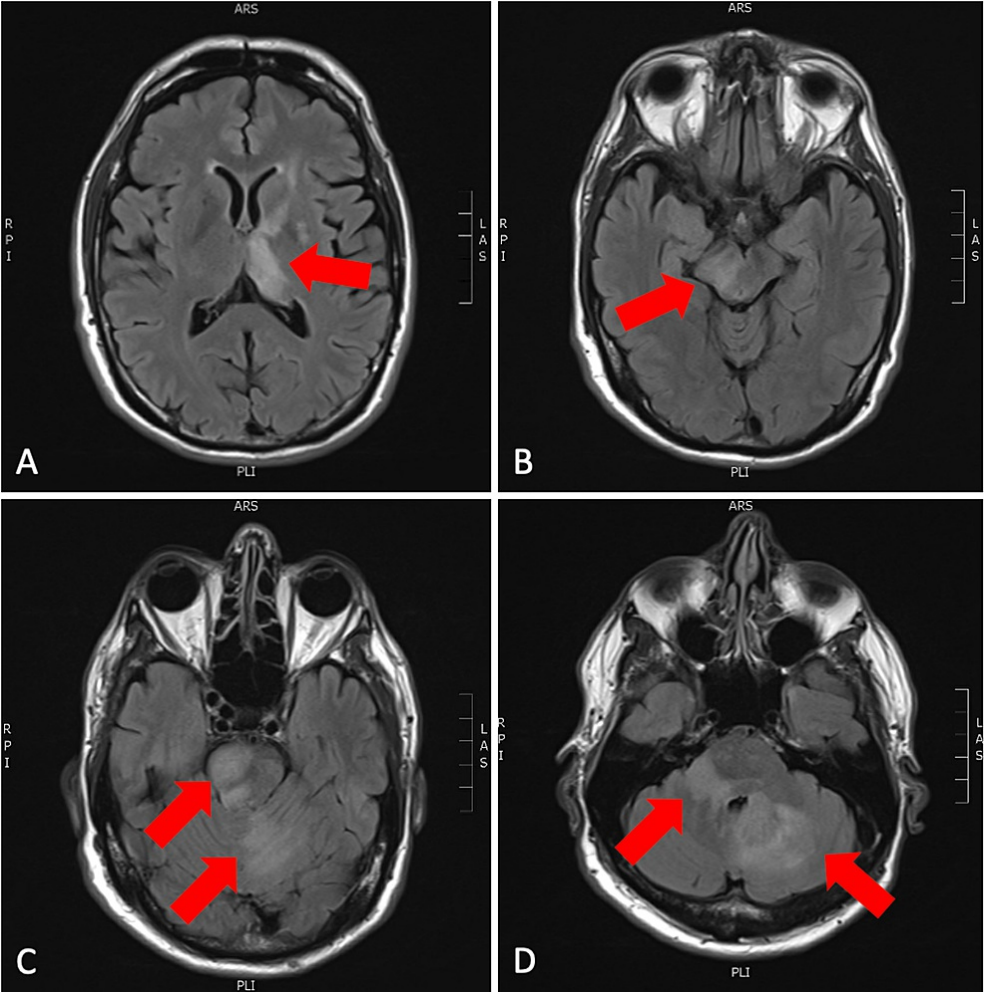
Axial slices from T2 FLAIR sequence of MRI brain with and without contrast. (A) Left thalamus and internal capsule involvement; (B) right midbrain involvement; (C) right pons and left cerebellar involvement; (D) bilateral cerebellar involvement.
